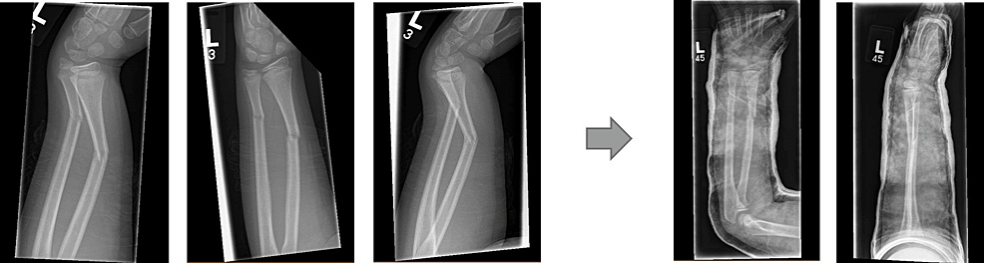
Radiograph 1 showing distal radius/ulna fracture in AP/Lat views and the subsequent manipulated views AP: Anteroposterior; Lat: Lateral
